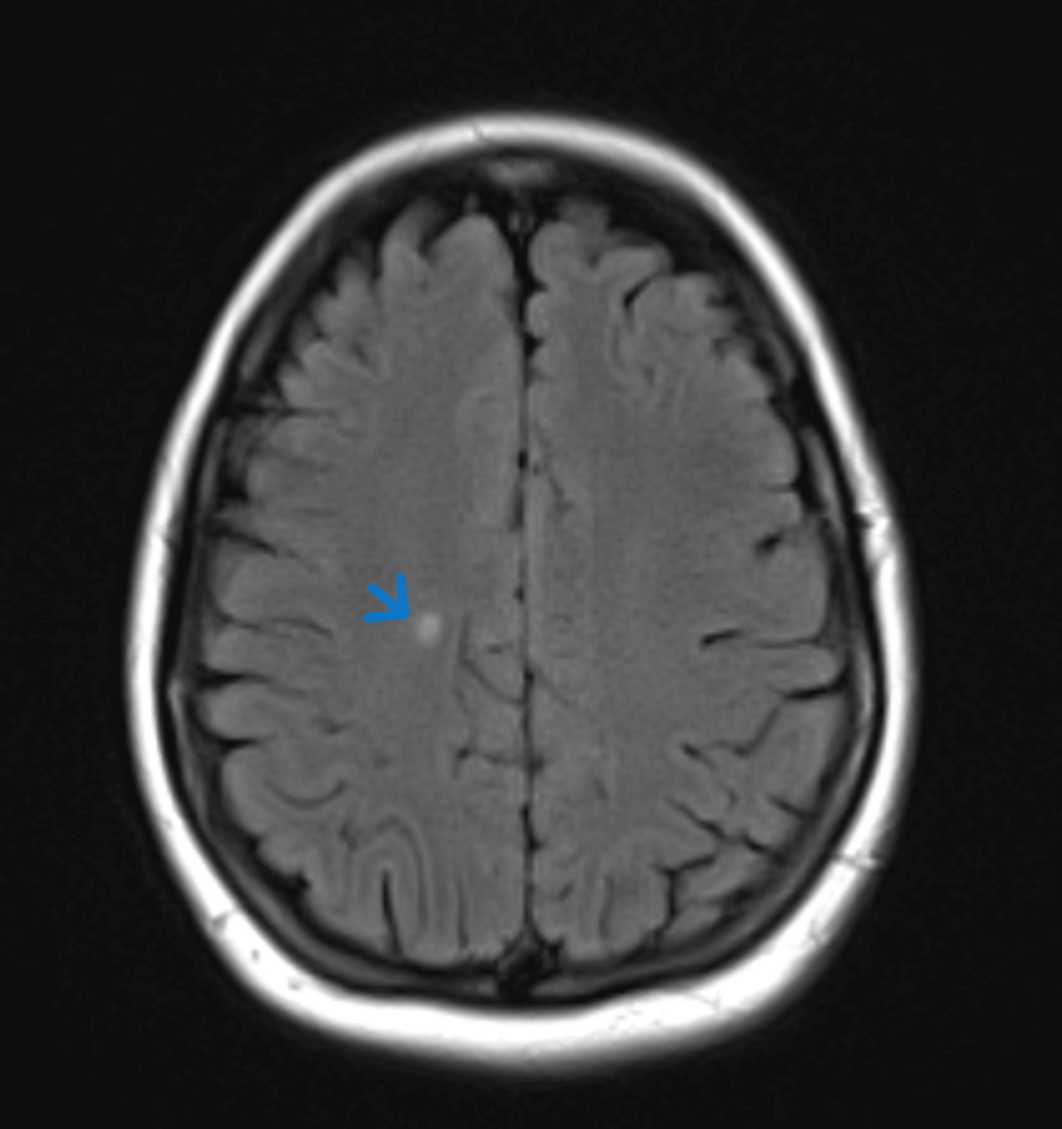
T2-weighted MRI of the brain showing a hyperintense focal lesion in the right centrum semiovale (indicated by the blue arrow), consistent with demyelination
